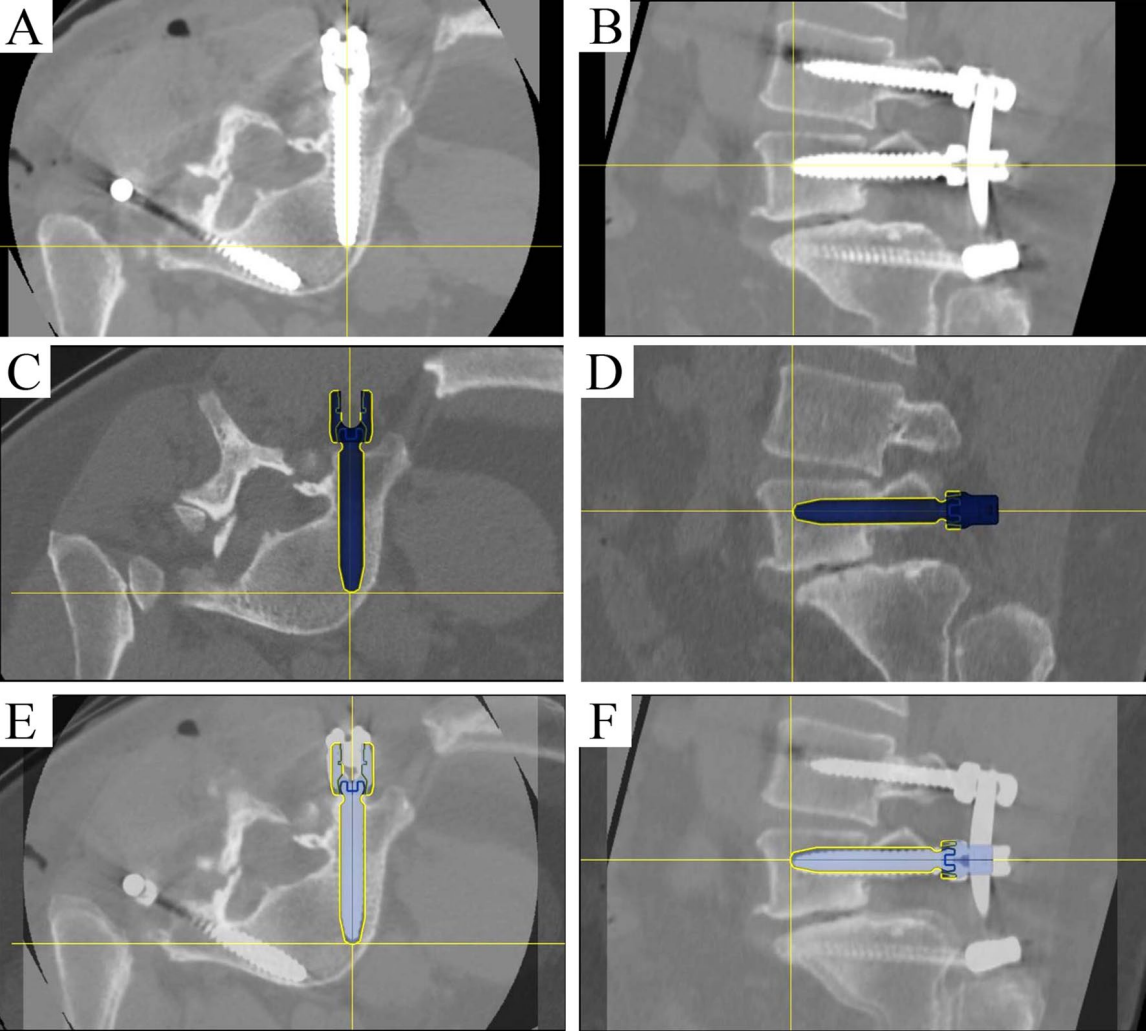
Screw tip, tail, and angle offset assessment. Postoperative CT of L5 screw placement without a medial or lateral breach in A axial and B sagittal planes. Right L5 screw planning in C axial and D sagittal planes. Image overlay analysis with preoperative planned trajectory and postoperative screw placement in E axial and F sagittal planes. The crosshairs indicate screw tip. *Note* This image is the property of the authors, who are the owners of its copyright
